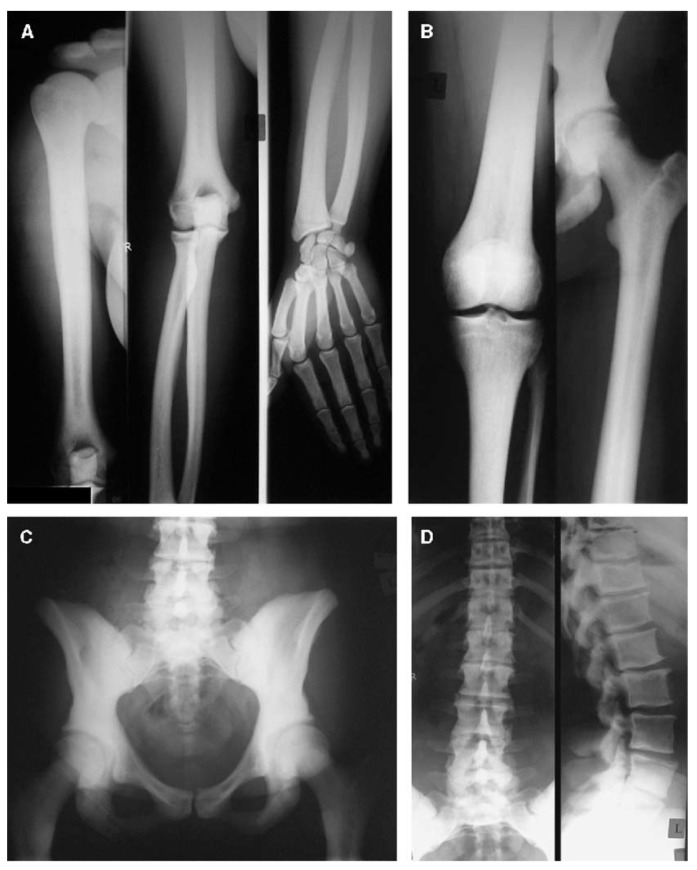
Radiographs of the upper extremity (A), lower extremity (B), pelvis (C), and spine (D) in a 31-year-old female with a gain-of-function variant in *LRP5*. Sclerosis can be seen in the vertebrae, the pelvic bones, the sacrum, and the cortices of the long bones, with corresponding narrowing of the medullary canals. Adapted with permission from Ref. [54]. 2005, *Journal of Bone and Mineral Research*.
